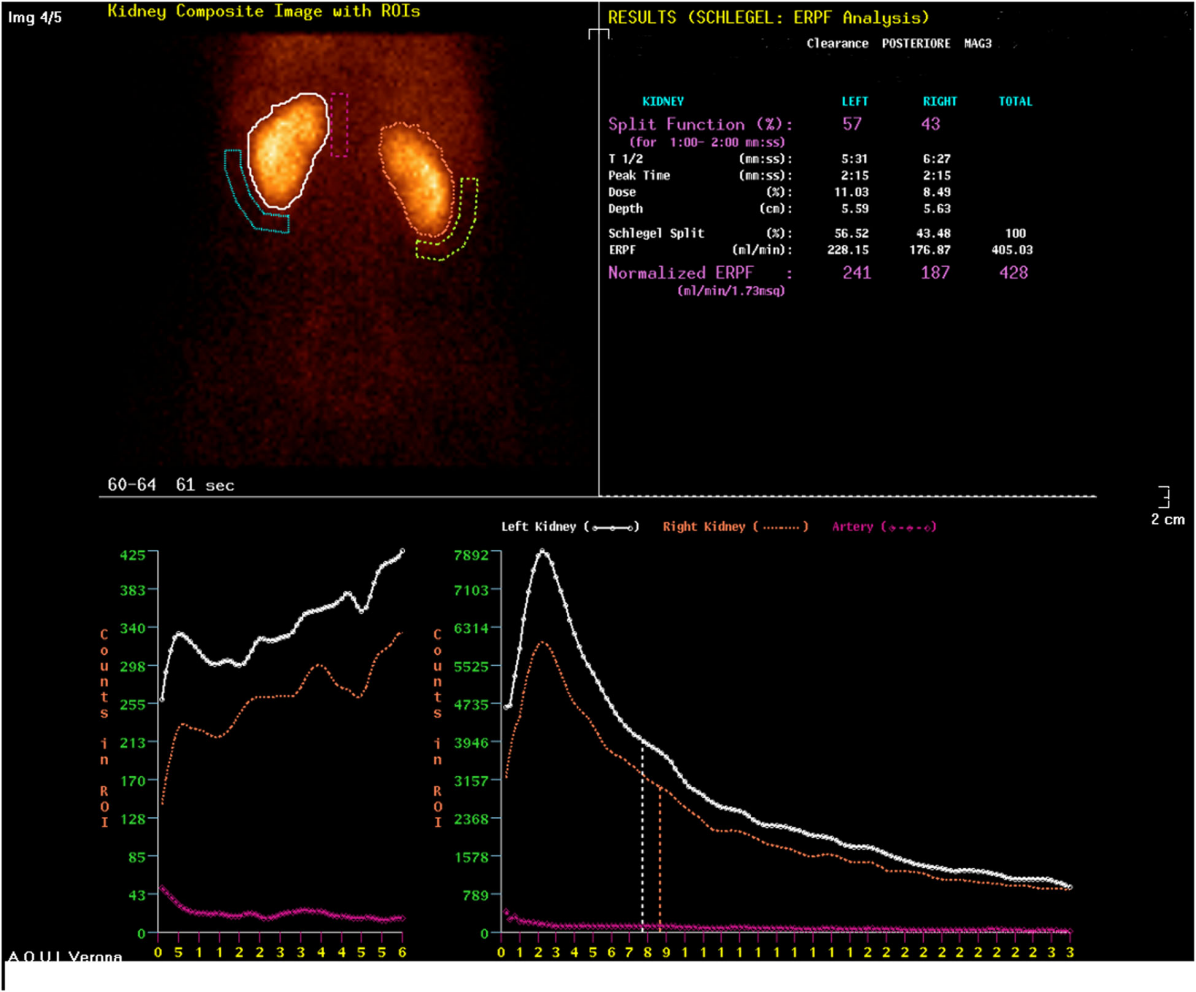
Evaluation of split renal function of the living kidney donor using conventional posterior Tc99m-mercaptoacetylgtriglycin (MAG3) scintigraphy. Right Kidney: 43%. Left Kidney: 57%
